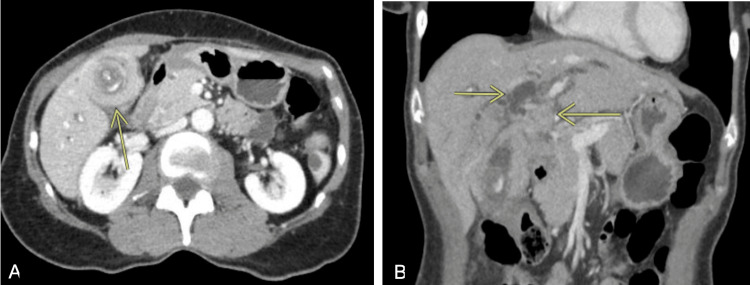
Initial CT demonstrating a large mass in the fundus of the gallbladder (A) with involvement of the liver, porta hepatis and intraductal biliary dilation (B).
